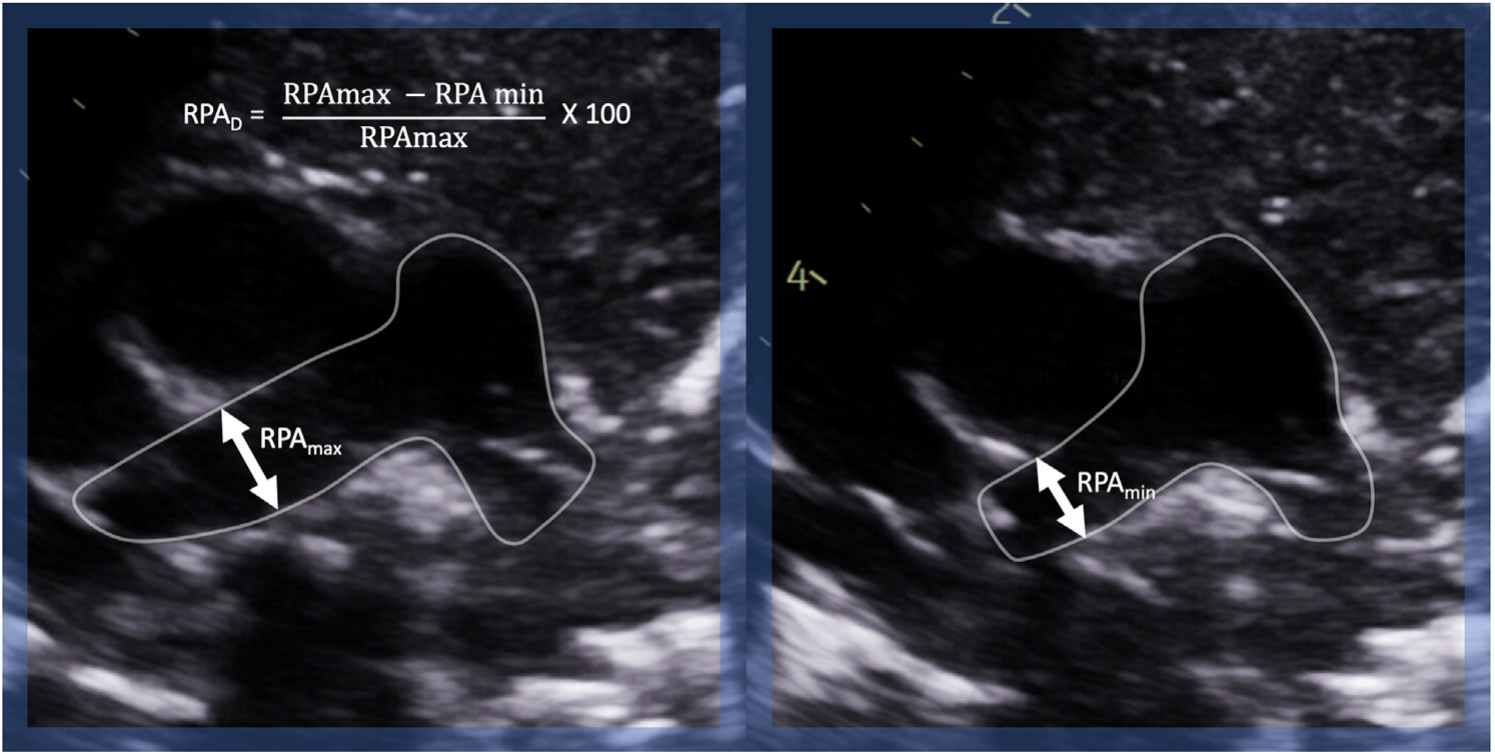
Right pulmonary artery distensibility (RPA_D_) index measurement in a parasternal short-axis view of the pulmonary arteries. max, maximal measurement; min, minimum measurement.
